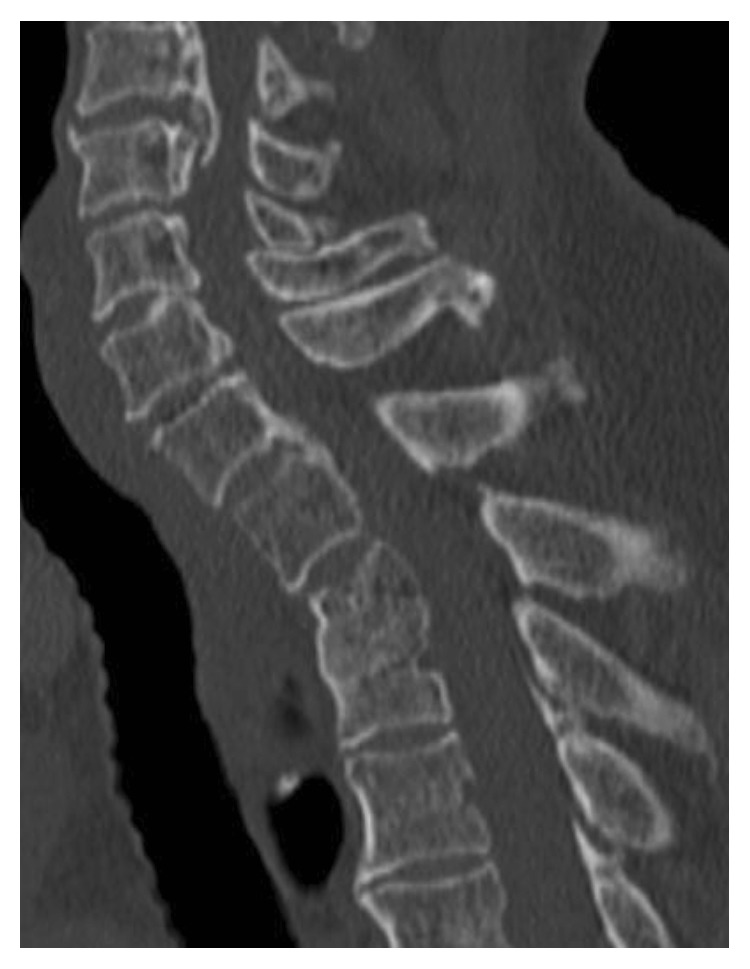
Sagittal CT of the cervicothoracic spine six months after operation shows bony fusion at T2/3.
